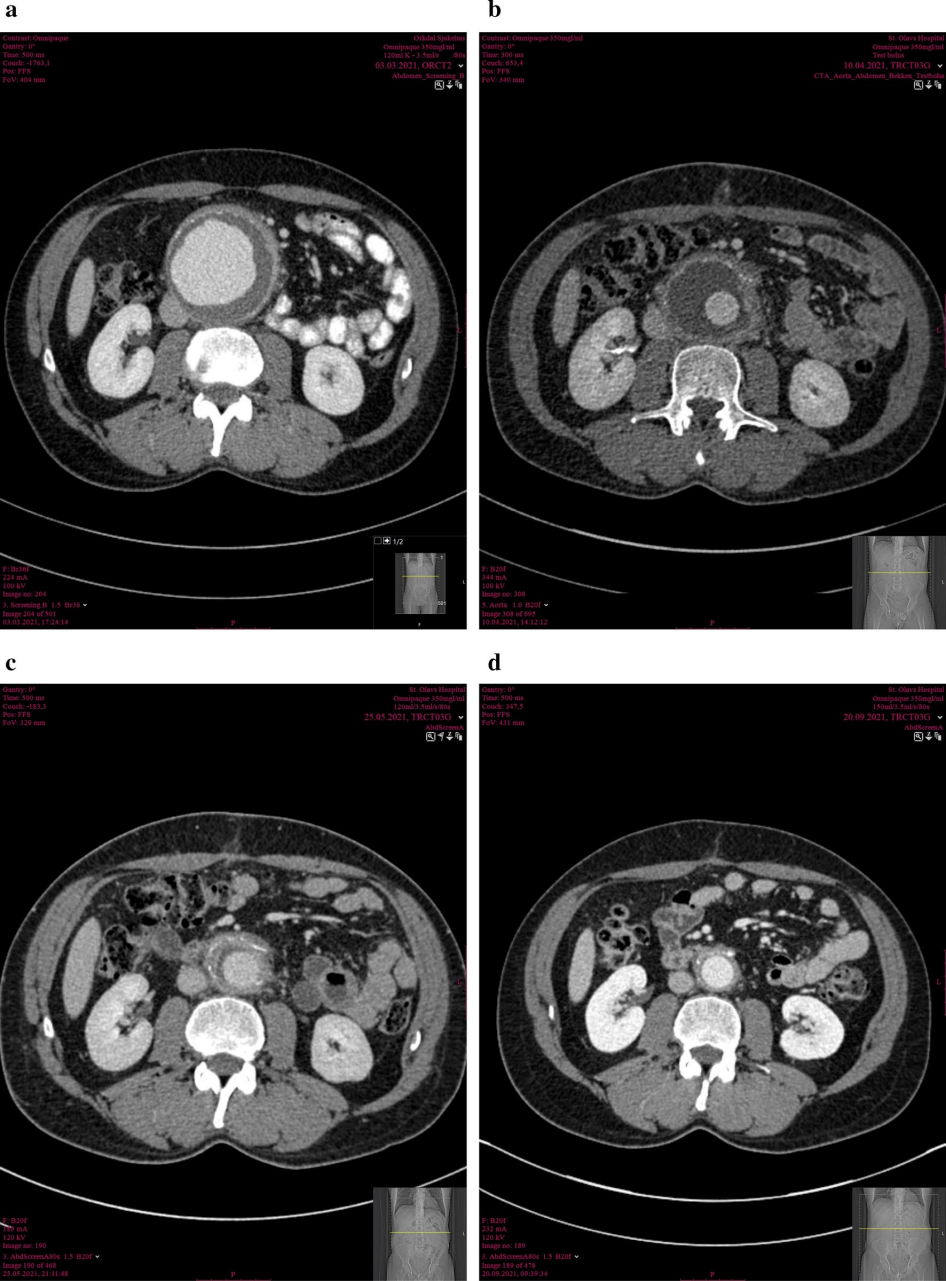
A–D Radiologic clinical timeline: Axial computed tomography angiograms of abdominal aortic aneurysm on admission (A), 1 month (B), 3 months (C), and 6 months (D) follow-up
